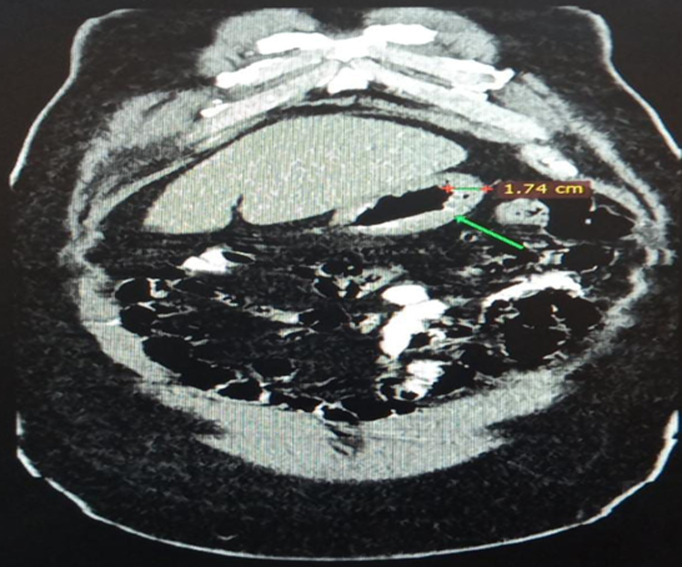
coronal view of abdominal CT scan showing gastric wall thickening
